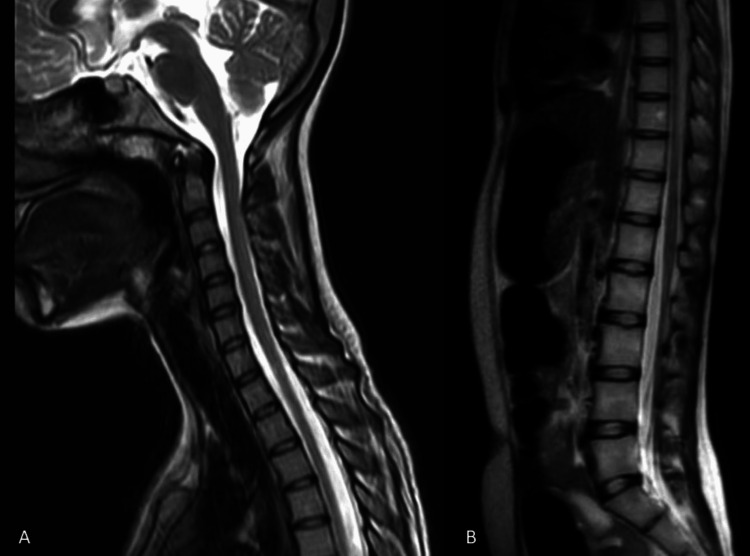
Normal MRI of cervical spine (A) and MRI whole spine screening (B) MRI: Magnetic Resonance Imaging
